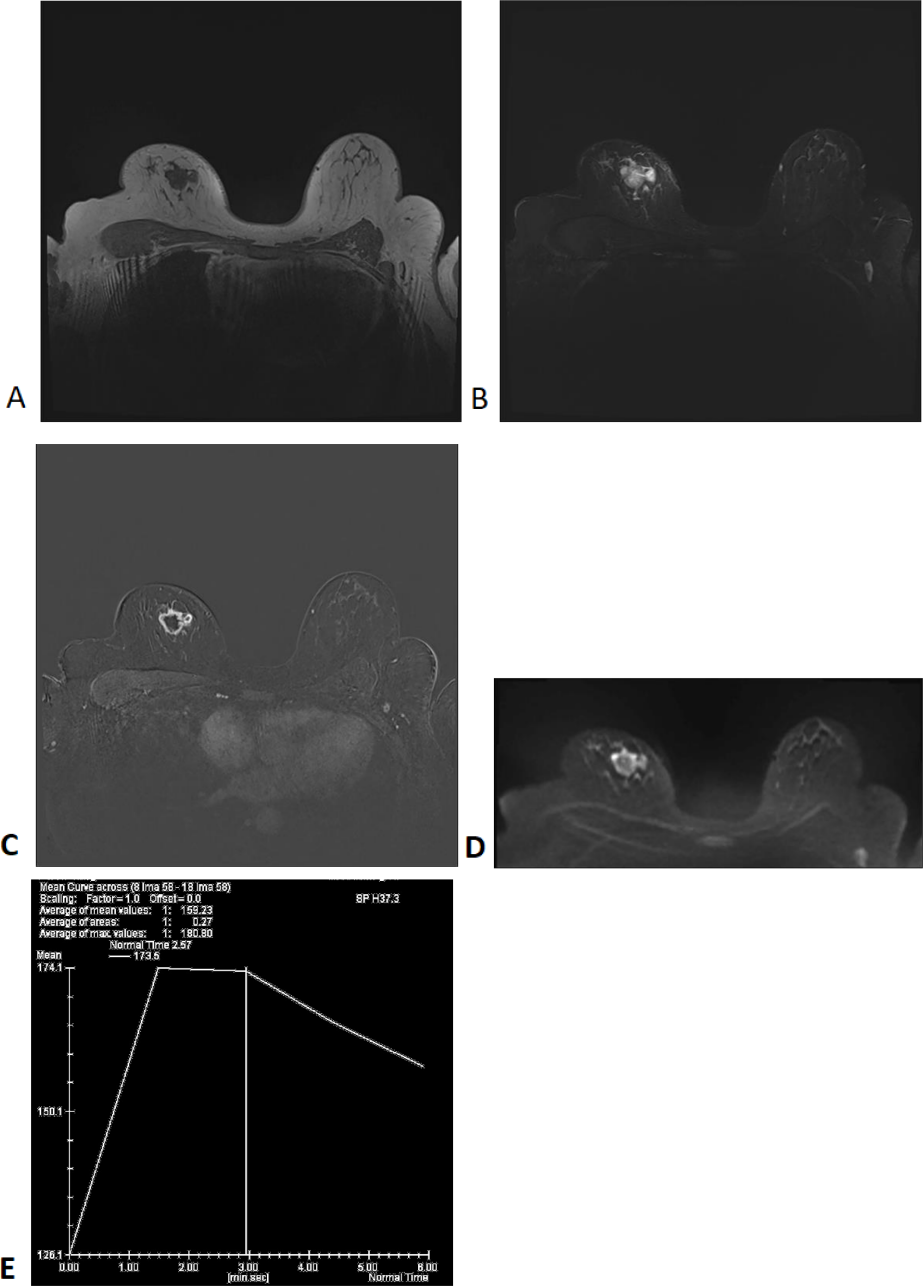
MRI Findings: T1WI (A), T2WI (B), T1WI+Contrast (C), DWI (D), DCE-Curve (E).
